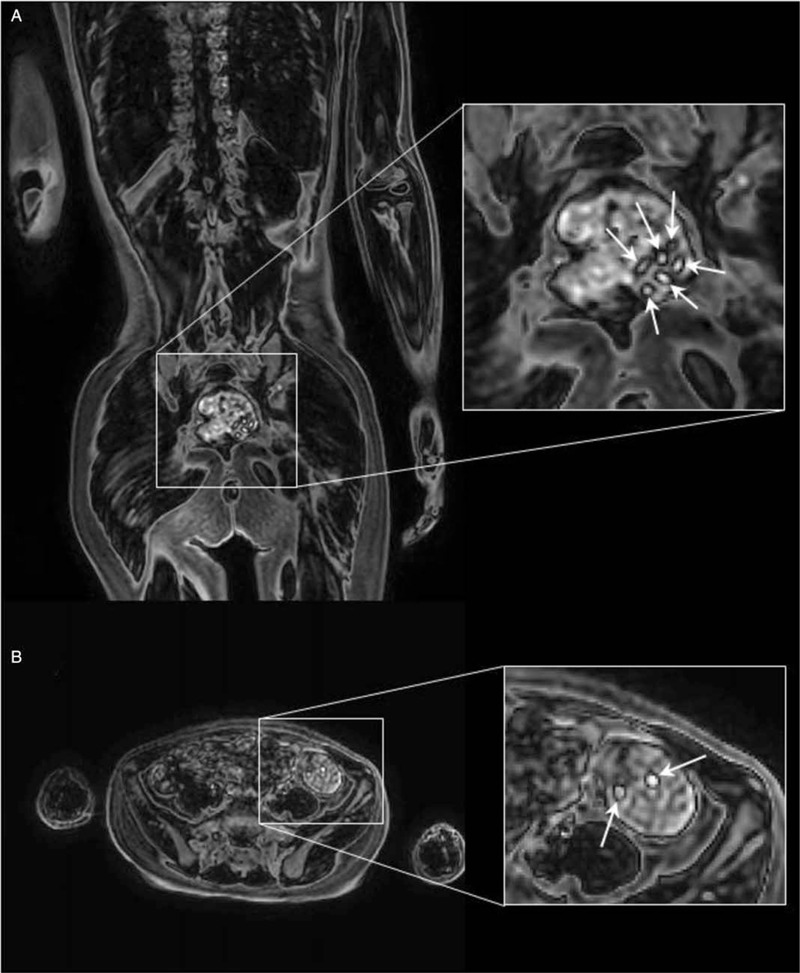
A, A coronal, derived in-phase minus out-of-phase magnetic resonance imaging (MRI) image of a 7-year-old patient participant, showing in the sigmoid/rectum 6 mini-capsules with positive signal against the chime. The mini-capsules are indicated by the white arrows in the corresponding expanded area on the right hand side. B, An axial, derived in-phase minus out-of-phase MRI image of the same patient participant, showing in the descending colon 2 mini-capsules with positive signal against the chyme. The mini-capsules are indicated by the white arrows in the corresponding expanded area on the right hand side.
